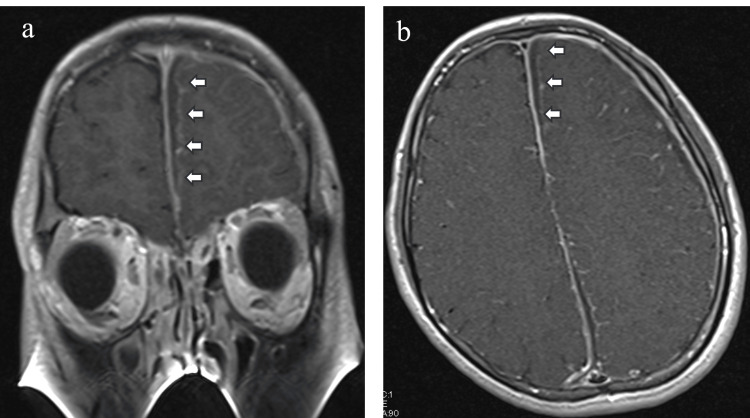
T1-Weighted Gadolinium Contrast Images a) Coronal MRI, b) axial MRI Uniform low-signal areas with contrast effect on T1-weighted gadolinium contrast images were observed under the dura mater along the left interhemispheric fissure.
